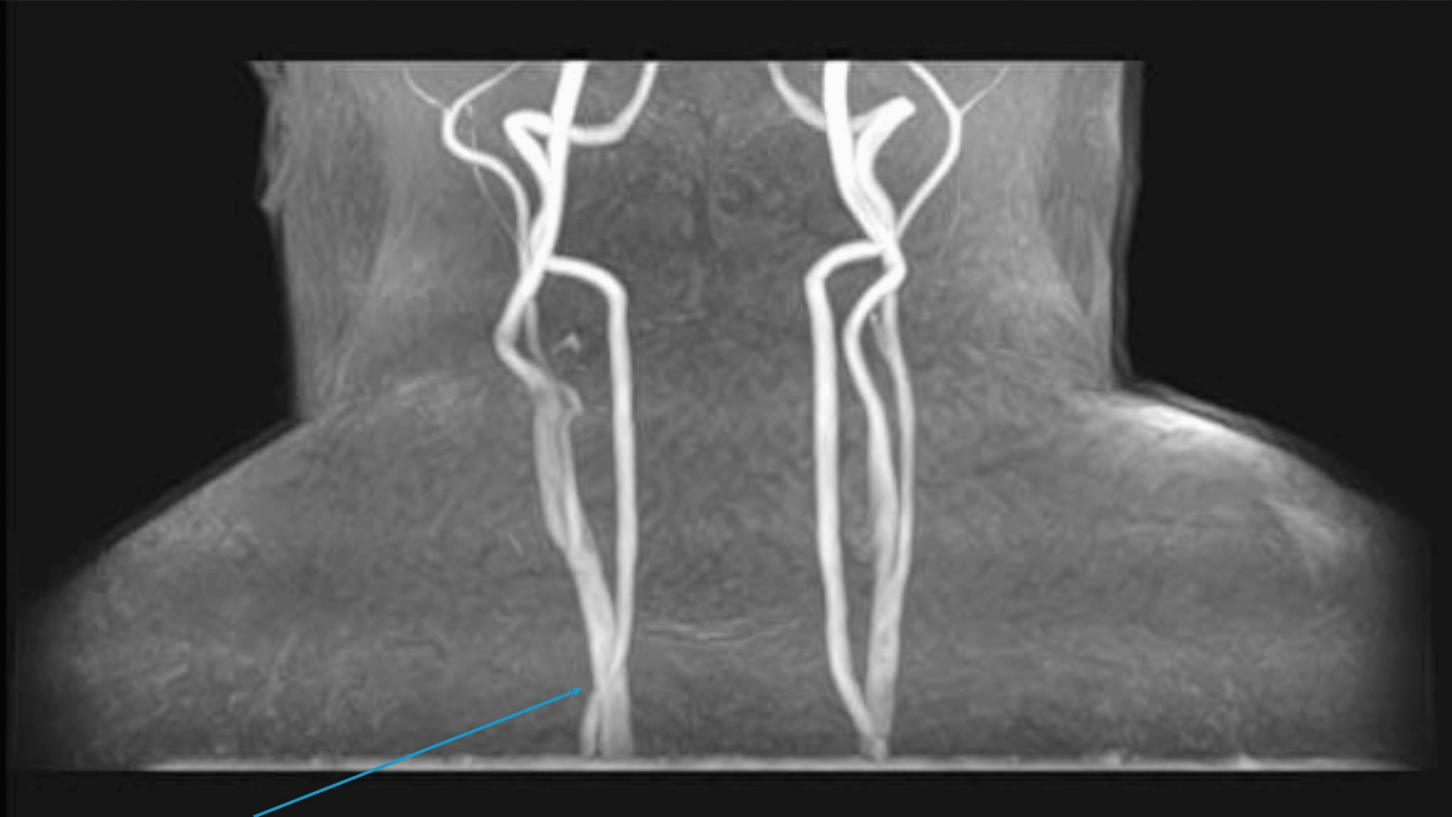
Magnetic resonance imaging of the carotids: radiological suggestion of significant eccentric short segment narrowing of the right internal carotid artery (blue arrow)
